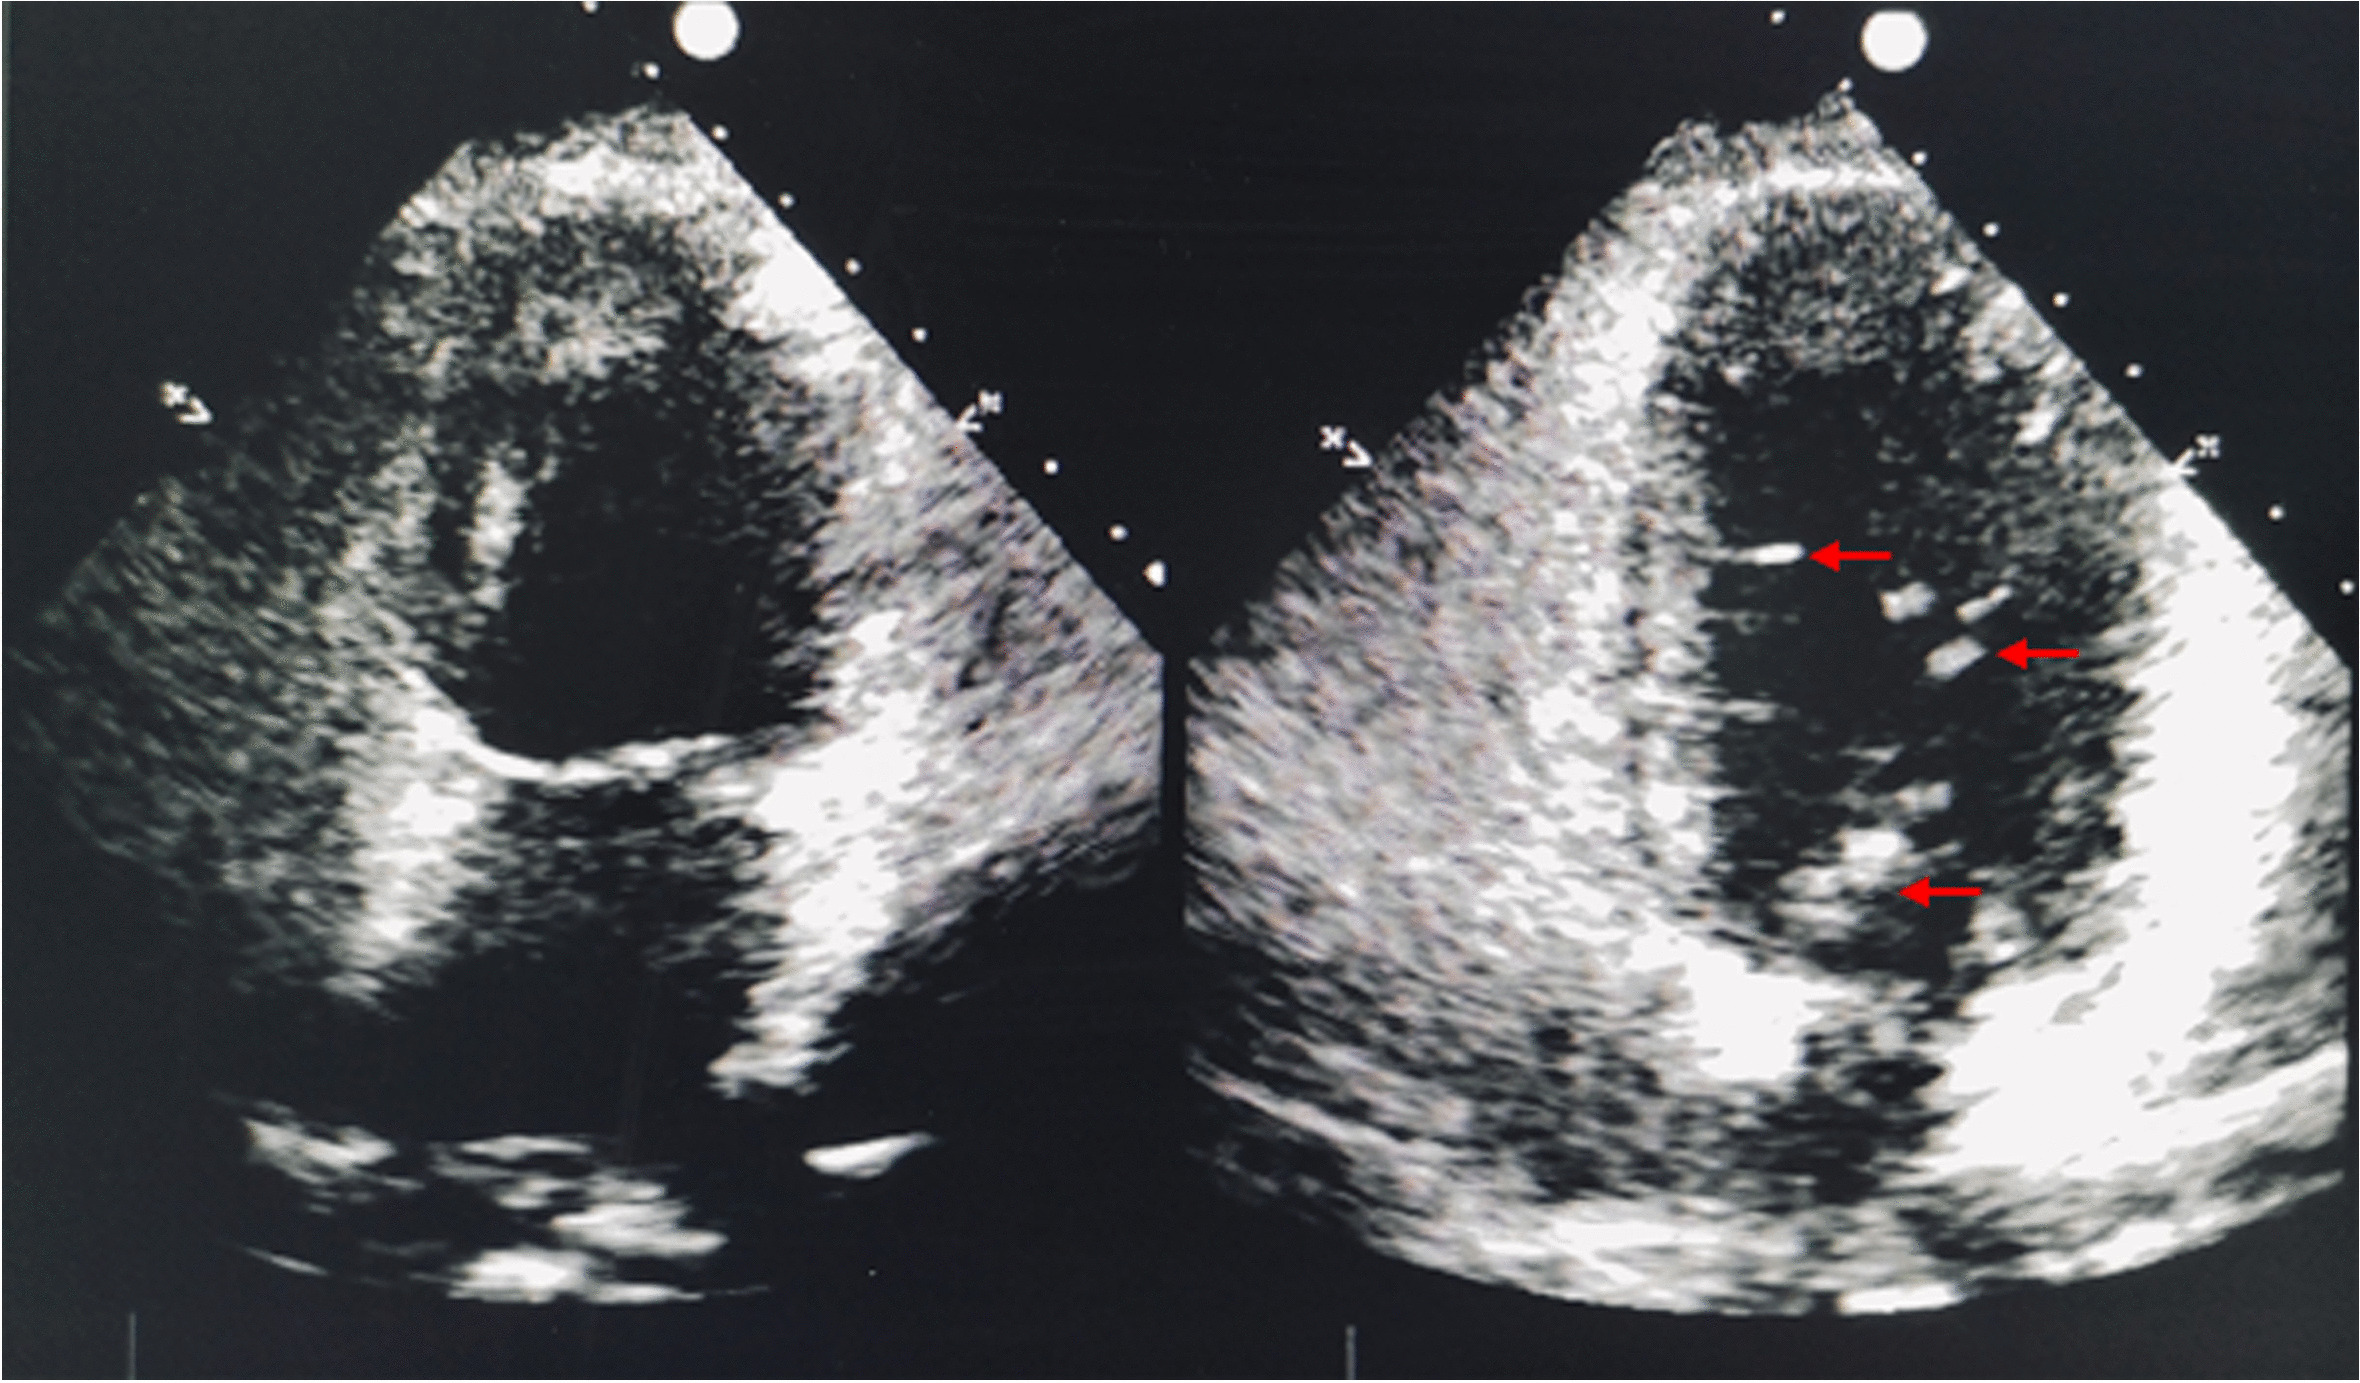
Contrast echocardiography of right ventricle indicated that a small amount of microbubbles (5–6/frame) could be detected in the left heart in the sixth cardiac cycle after Valsalva maneuvers. Microbubbles are noted with arrows
